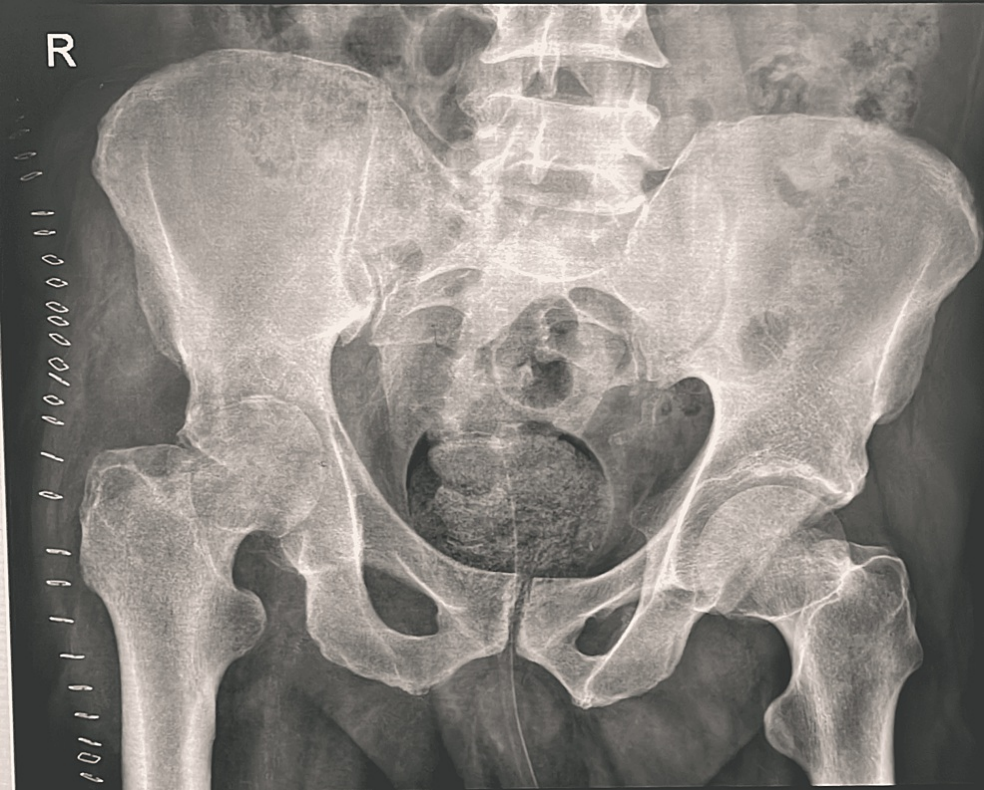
Pelvic x-ray showed severe right hip arthritic changes.
